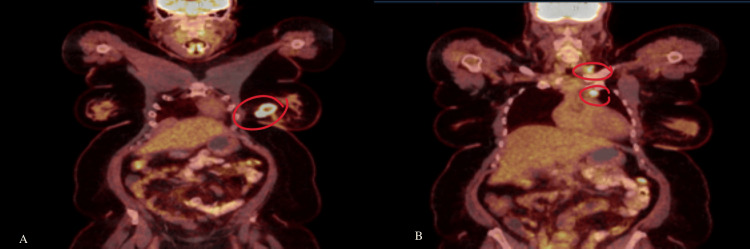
PET scan PET scan of the patient’s chest with a more exterior slice on the left and an interior slice on the right. A: highlights an increased FDG uptake in the left breast; B: shows an increased FDG uptake in a supraclavicular lymph node (2.1 SUV) and an infraclavicular lymph node (2.2 SUV). Both are circled in red. FDG: fluorodeoxyglucose; SUV: standardized uptake value
